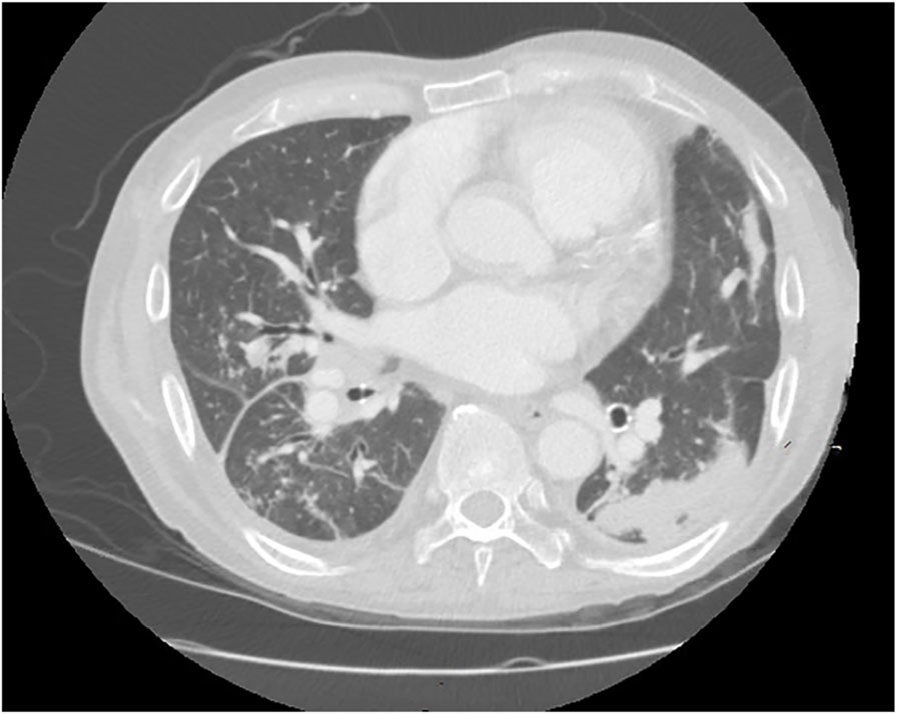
CT chest with no contrast showing multifocal pneumonia
